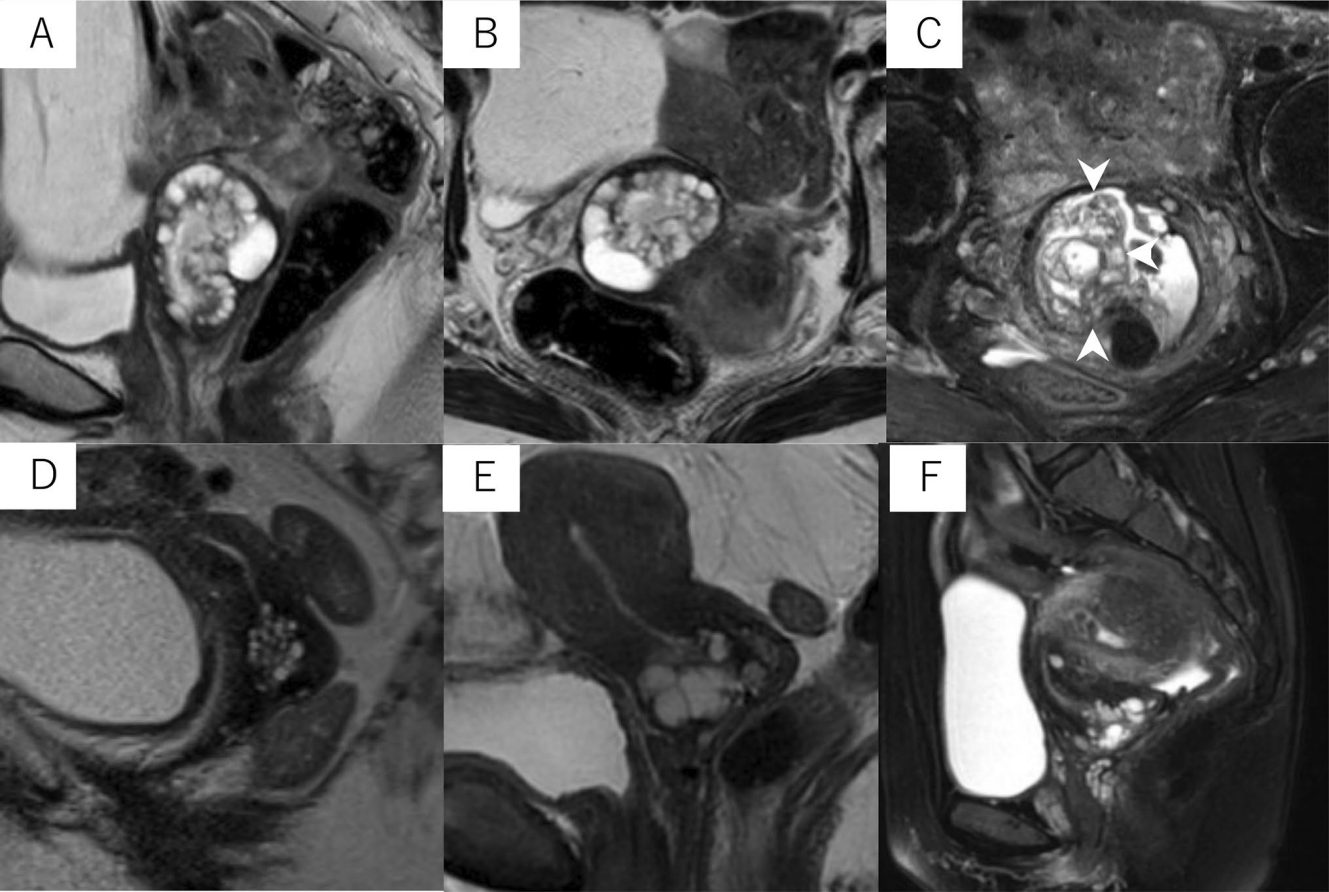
Five types of magnetic resonance imaging features. A,B Typical cosmos pattern. Sagittal and axial T2-weighted images (T2WIs) shows that the cystic aggregate lesion is mainly located in the upper part of the cervix. Small cysts (< 5 mm) and solid components are visible in the central part of the lesion, surrounded by relatively large cysts (≥ 5 mm). The solid components in the central part show hypointensity similar to that of the cervical stroma or slight hyperintensity compared to the cervical stroma, and are located between the cysts. C Atypical cosmos pattern. This lesion meets the criteria for the typical cosmos pattern, with small cysts arranged on the endocervical side and relatively larger cysts on the cervical stromal side. However, it is a polypoid lesion protruding from the right side of the cervical stroma into the endocervical canal (arrowhead). D Microcystic pattern. Sagittal T2WI shows that the lesion is located in the upper part of the cervix and composed of many tiny aggregated cysts. E Macrocystic pattern. This lesion is mainly made of relatively large cysts on sagittal T2WI. F Other pattern. This lesion is composed of variably-sized cysts near the external cervical os
